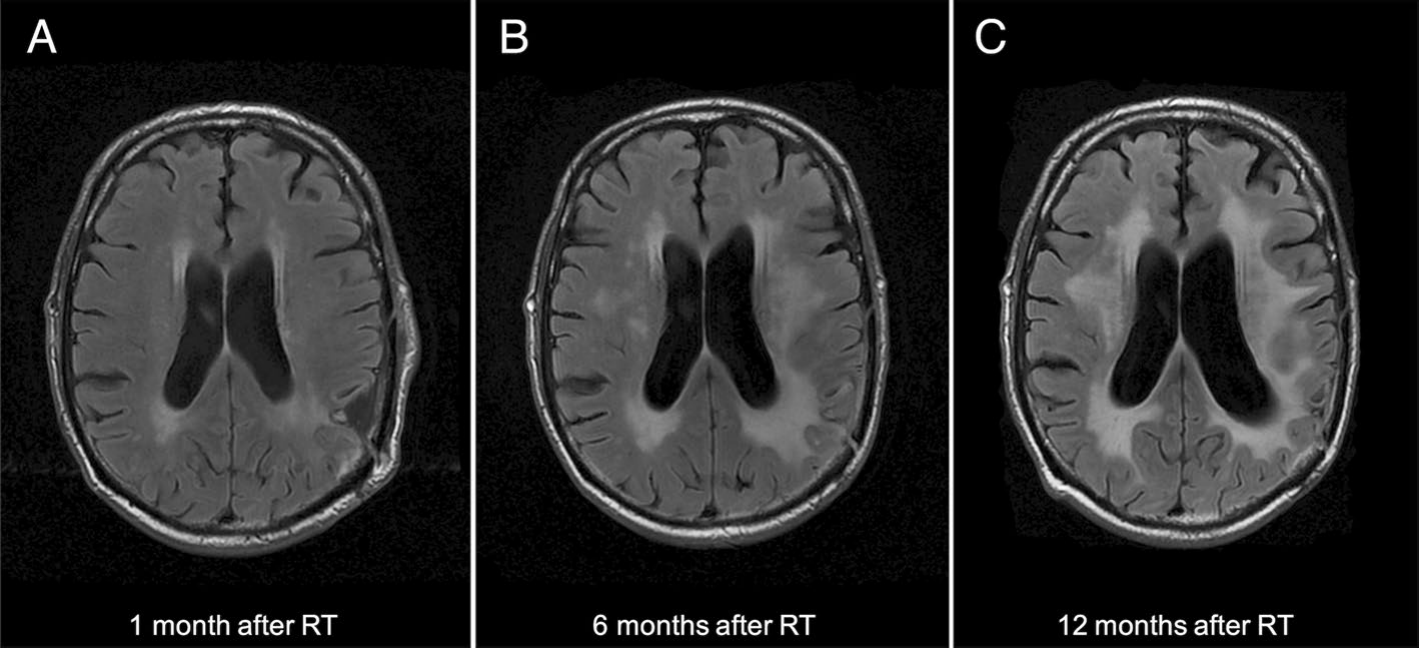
MRI findings of radiation-induced neurotoxicity presenting as rapidly progressing white matter hyperintensities associated with cortical and subcortical atrophy. The images show MRI findings at 1 month (A), 6 months (B) and 12 months (C) after RT. The patient received 60 Gy in 30 fractions with concomitant TMZ for a left parietal glioblastoma. After 1 year of follow up, memory deficits were present (MOCA 13/30) as were gait difficulties and urinary incontinence. The patients had the rs2120825 risk allele T (TG).
